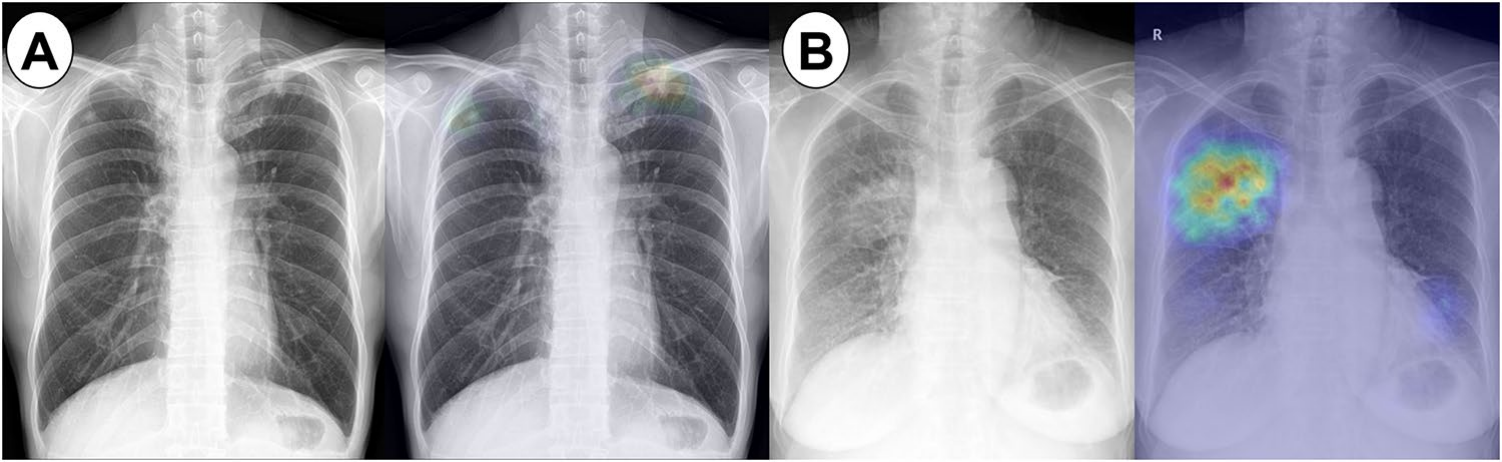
Representative chest X-ray images of patients with pulmonary tuberculosis. (A) Chest X-ray of a 48-year-old male patient with a tuberculosis extent score of 3.7. This patient was cured following a 6-month treatment course with the standard regimen*. (B) Chest X-ray of a 71-year-old female patient with a tuberculosis extent score of 16.8. The patient died during the course of the standard treatment regimen*. *Standard regimen refers to the combination of isoniazid, rifampicin, ethambutol, and pyrazinamide.
